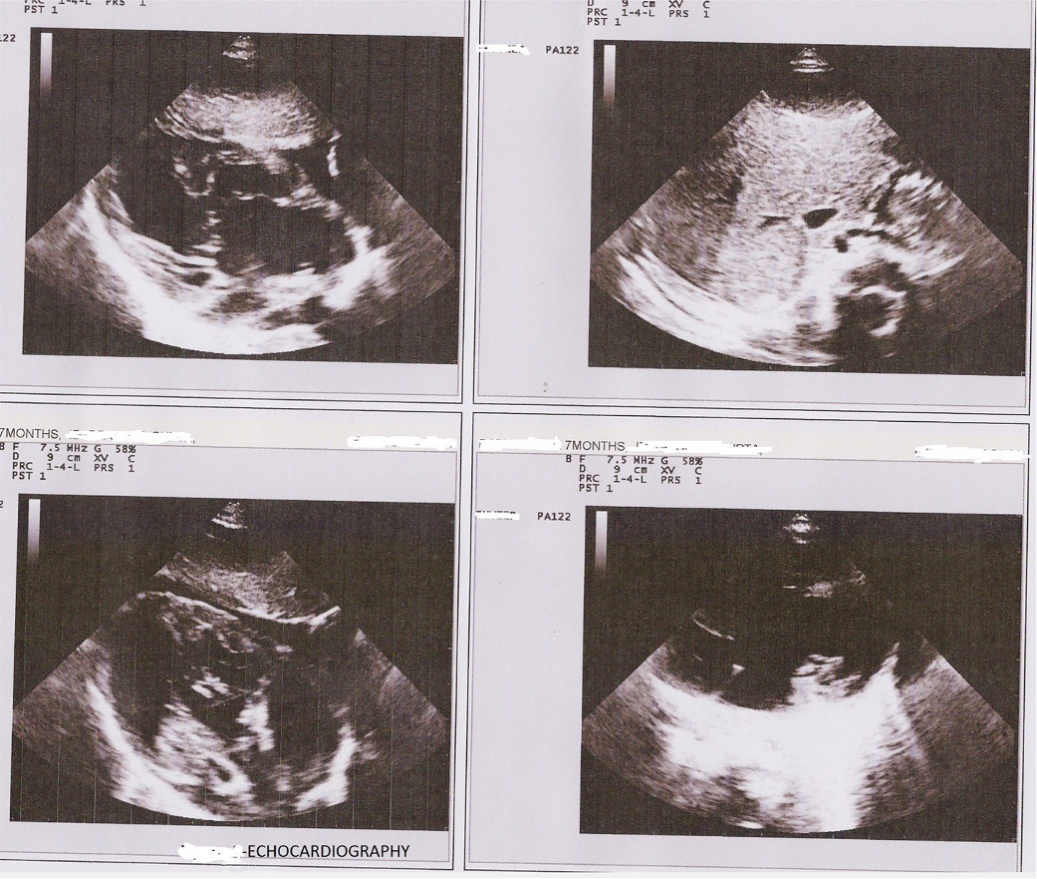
Echocardiography showing complex pattern of heart disease.
